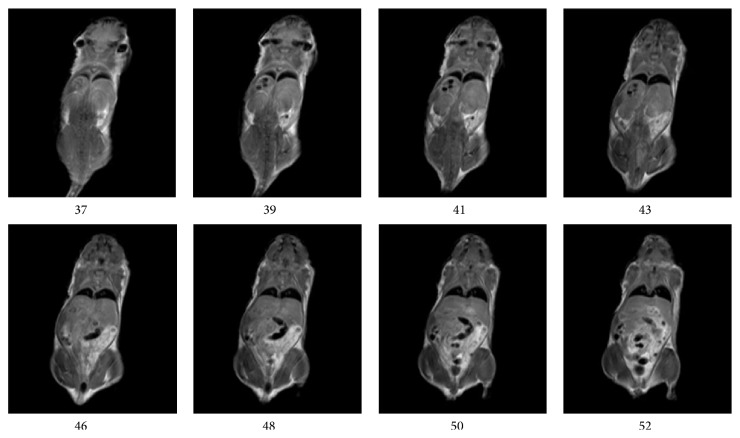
The 8 coronal T1-weighted images, all clear images from head to tail were clearly obtained (1.2 T, 60 mm).
